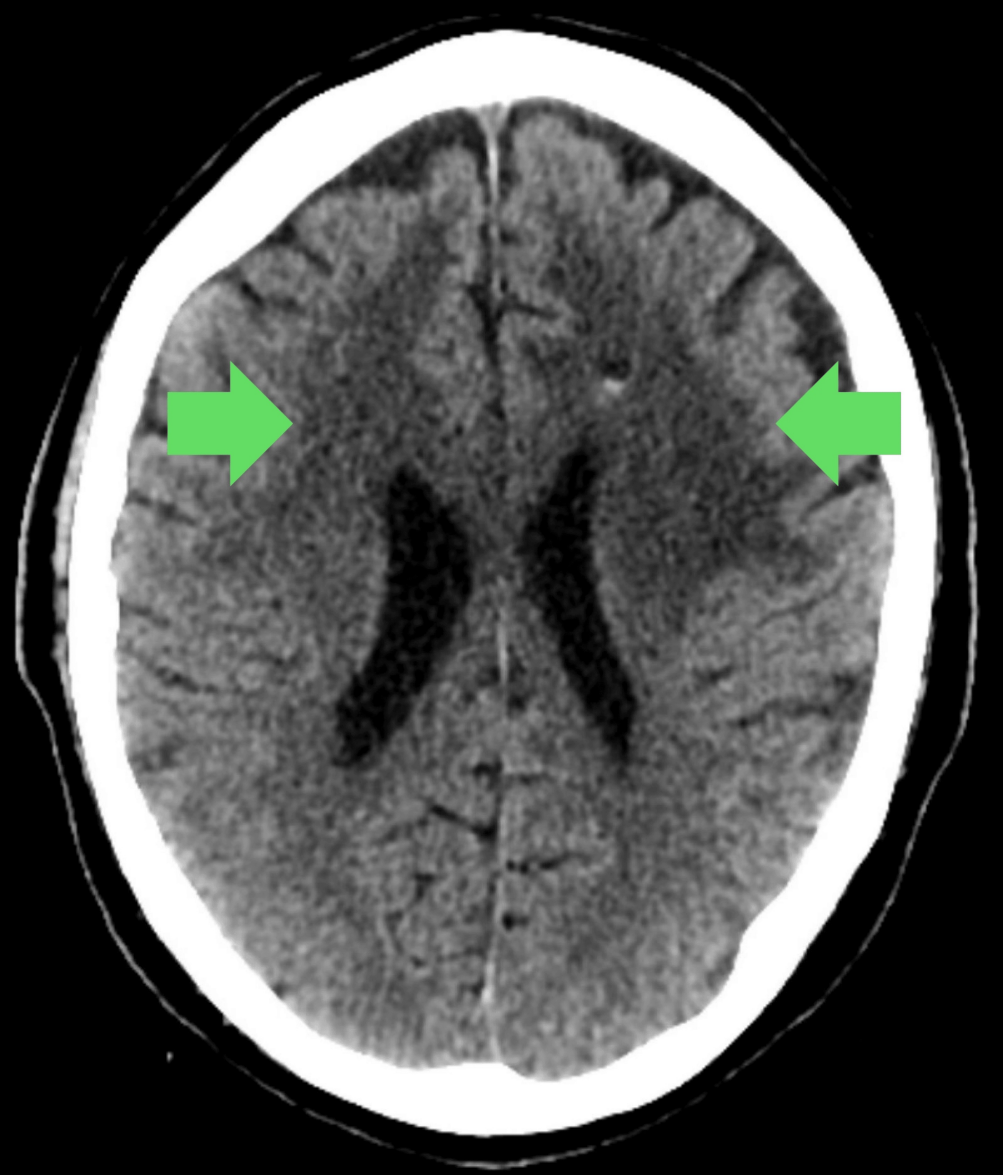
Head CT of patient 2 with RPCNSL Low-attenuation foci are present in the bilateral frontal lobes and centrum semiovale, crossing the midline. RPCNSL = recurrent central nervous system lymphoma
